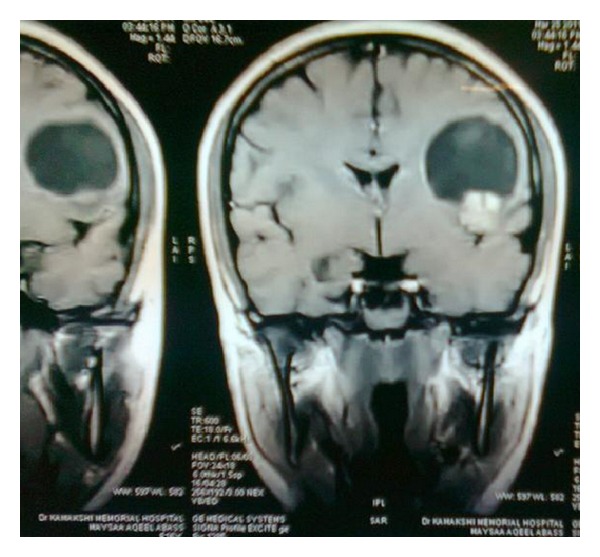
Plain MRI—coronal section showing lesion in the left frontoparietal region.
